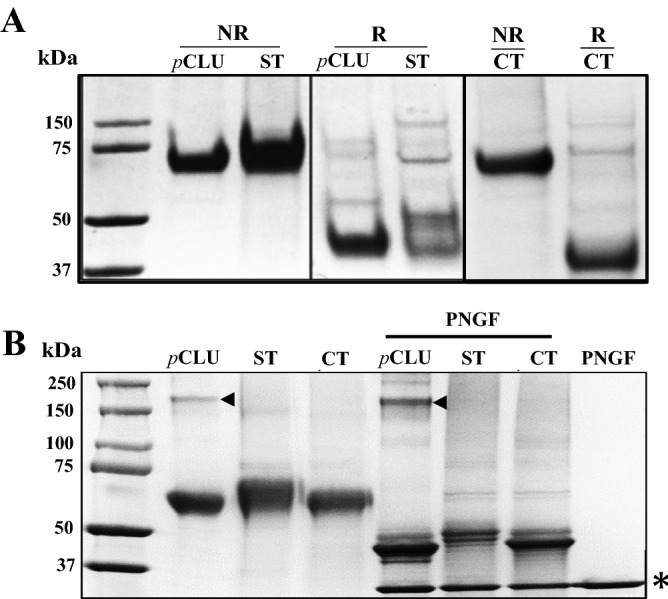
SDS PAGE analyses of *p*CLU and *r*CLU. SDS PAGE analysis of (A) purified *p*CLU, *r*CLU-αC-ST (ST) and *r*CLU-CT (CT) under non-reducing (NR) and reducing conditions (R), and (B) the same samples (non-reduced) treated with or without PNGaseF (PNGF). In (A) and (B), the molecular mass of protein standards (left lane) are shown in kDa. In (B), the position of a low level of contaminating human IgG that co-purifies with *p*CLU (visible on this gel) is indicated by black arrowheads, and the position of PNGaseF enzyme is indicated by an asterisk at the bottom right of the image. The very minor bands that under reducing conditions migrate to ~ 75 kDa or greater are likely to represent different glycoforms of a small fraction of *p*CLU/*r*CLU that is uncleaved when secreted. The results shown are each representative of two independent experiments. In (A), the black bordering rectangles surround images of discrete parts of gels. The complete gel images corresponding to the left and centre panels in (A), and the right panel, are shown in Supplementary Materials Figures S3B and S3C, respectively.
